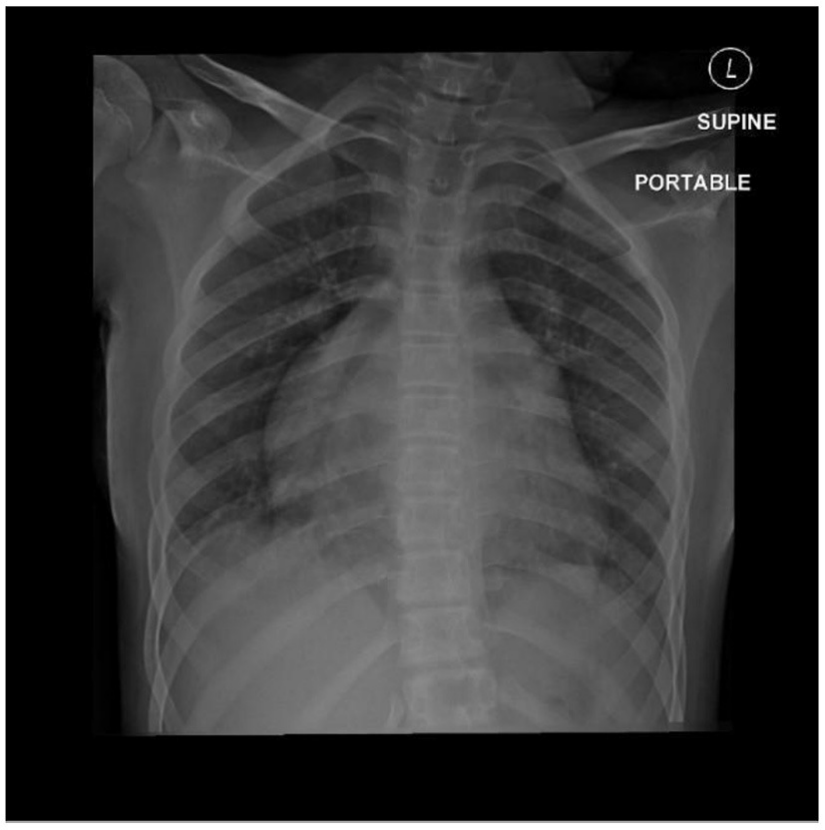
Chest x-ray showing cardiomegaly with a prominent atrium.
